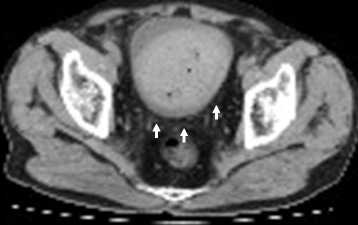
Computed tomography demonstrated that the bladder was filled with a blood clot (arrow)
